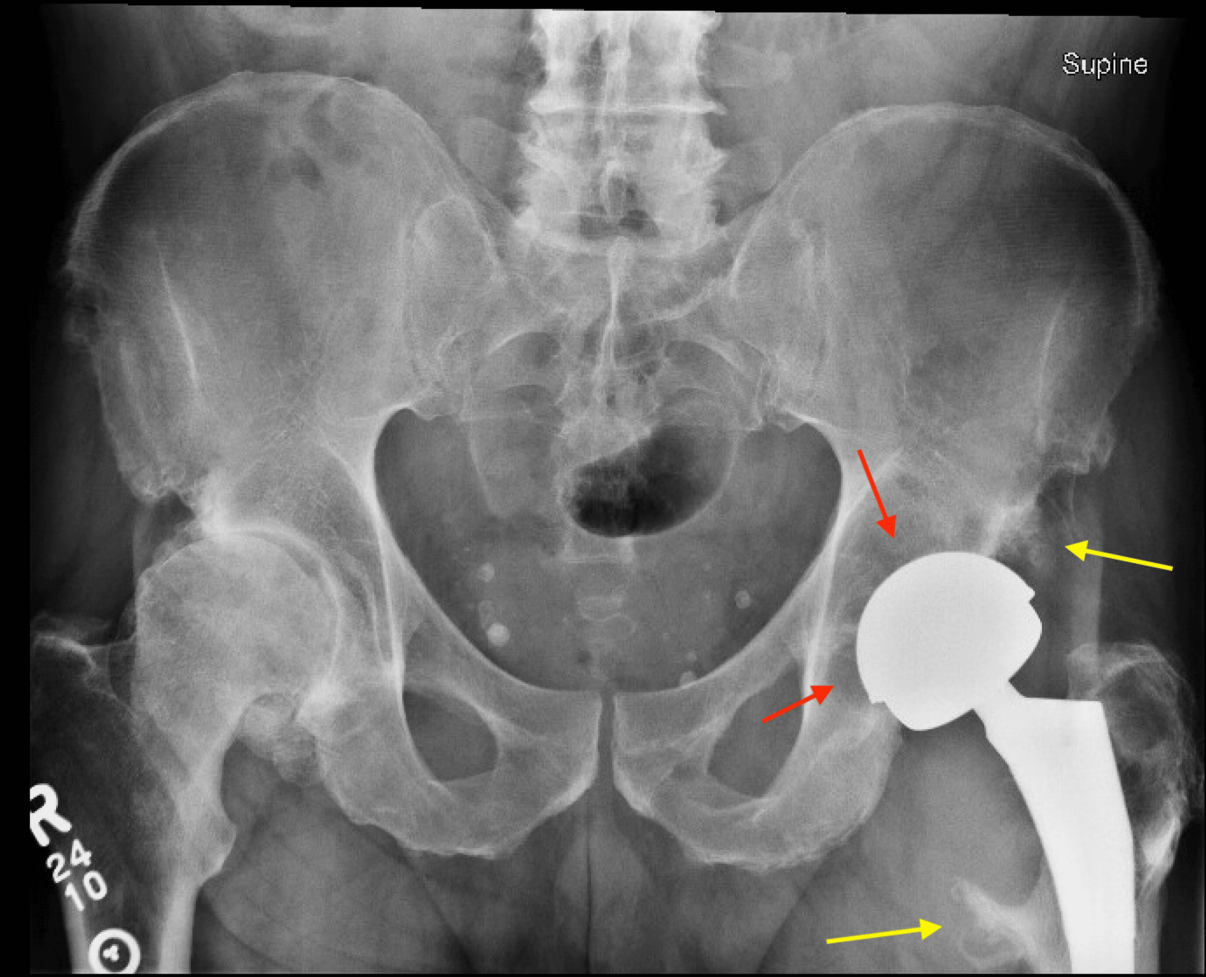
Anterior-posterior radiograph of the left hip demonstrating postsurgical changes consistent with prior metal-on-metal total hip arthroplasty. Red arrows: Patchy periprosthetic lucencies surrounding the acetabular component; Yellow arrows: Enthesopathic changes at the lesser trochanter and along the anterior inferior iliac spine.
